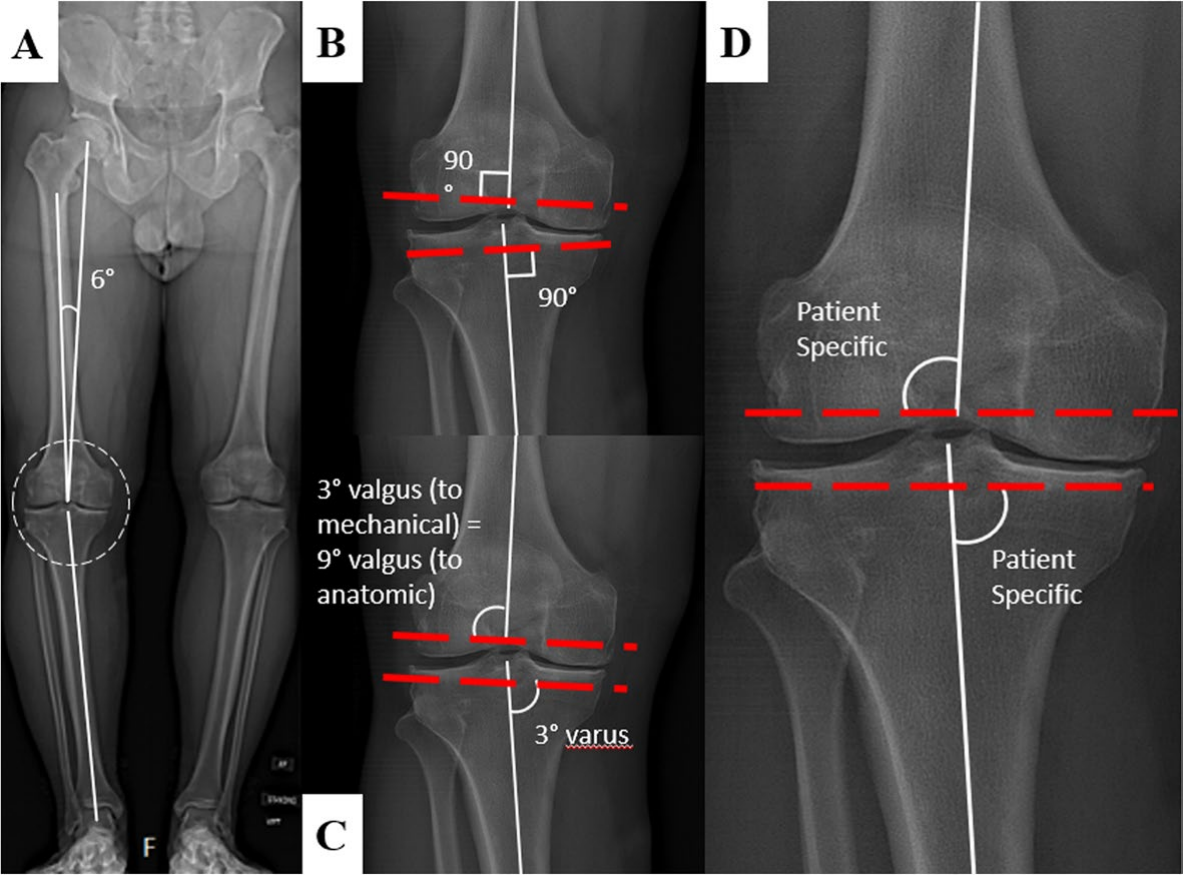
a Long-leg radiograph demonstrating the mechanical axis relative to the anatomical axis of the lower extremity. b Mechanical alignment of the knee places components at 90° with the mechanical axis of each bone. c Anatomical alignment aims for a lateral distal femoral angle (LDFA) of 3° valgus to the femoral mechanical axis (also commonly described as 9° valgus to the femoral anatomical axis). The tibial components are placed with a medial proximal tibial (MPTA) of 3° varus. d Individualized alignment techniques. Image reproduced from original work with permission from Dr. Jonathan Vigdorchik
